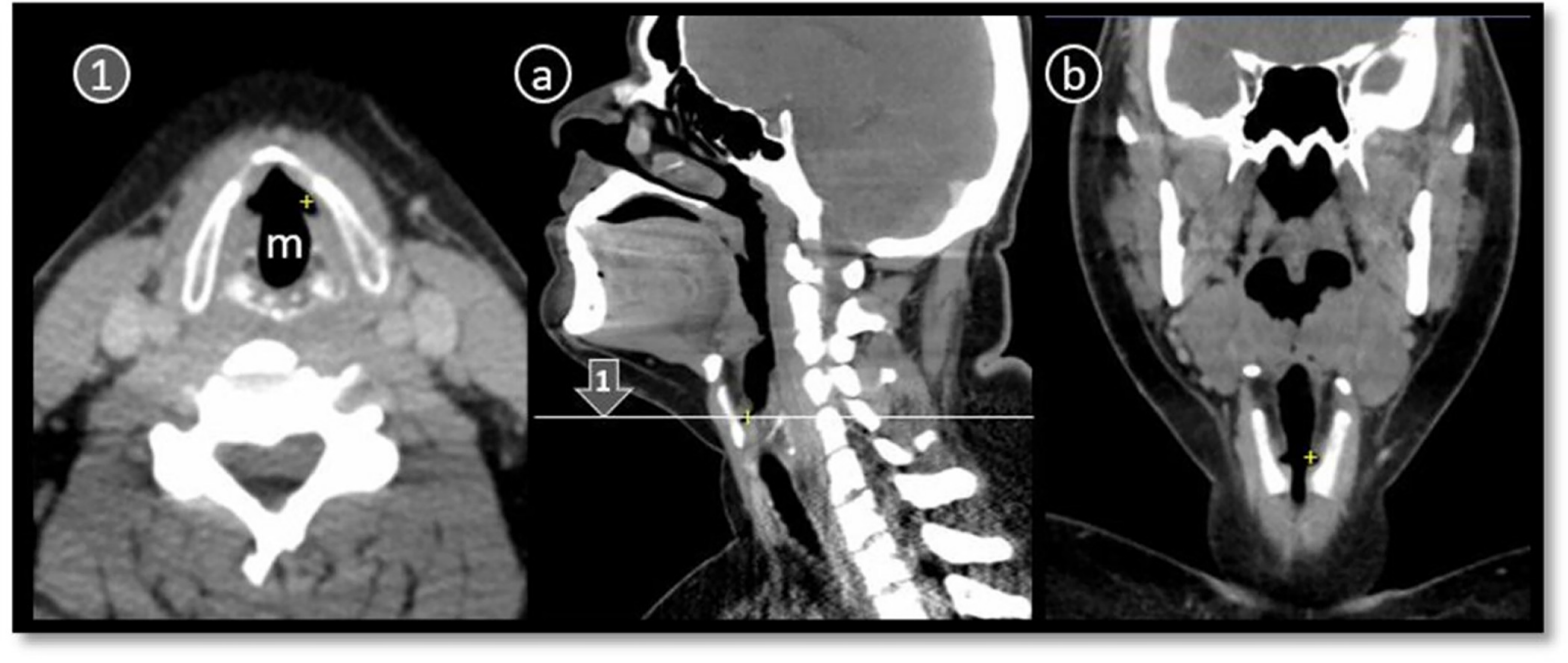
Post-contrast CT neck showing mushroom (m) shape of glottic lumen in non-parallel vocal cord (NPVC) plane① due to crossing of plane① (a) through laryngeal ventricle (+, a, b) rather than a true vocal cord. Left laryngeal ventricle is marked by + sign.
